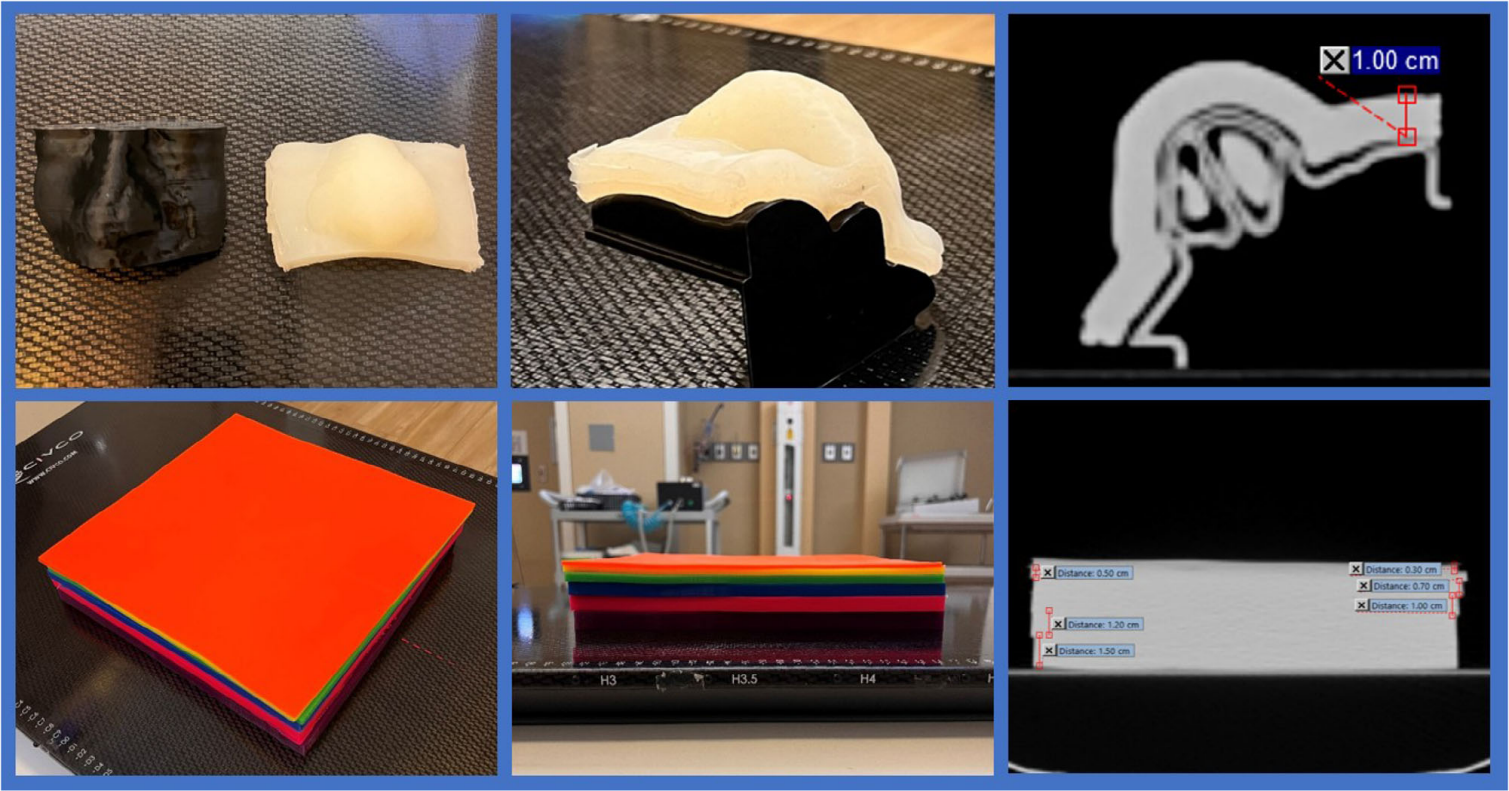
Examples of the bespoke (top) and color sheet (bottom) silicone bolus, and the corresponding QA setup on the CT scanner, with an example of the thickness measurement.
